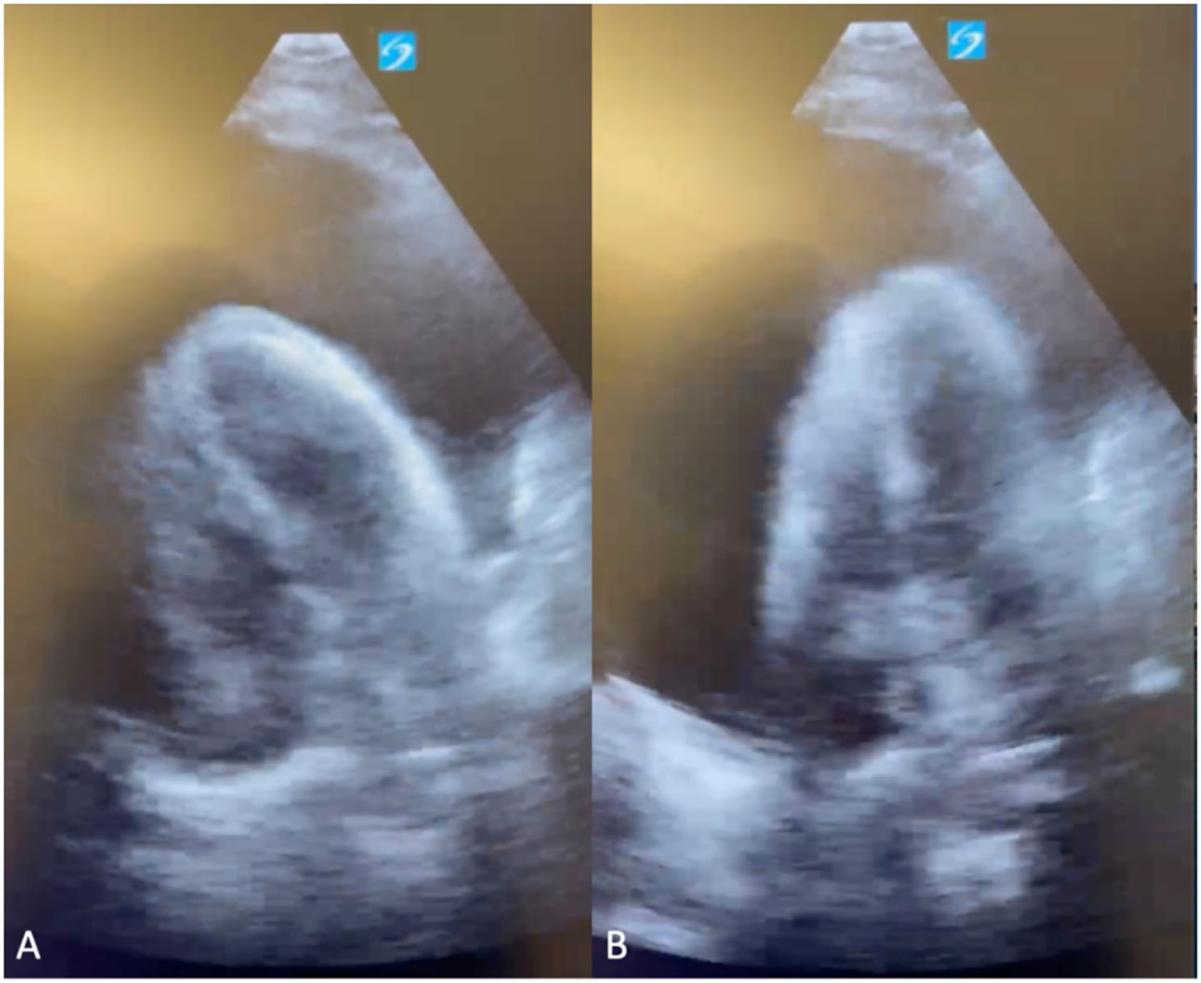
Point-of-care ultrasonography (POCUS) of large pericardial effusion. (A) POCUS demonstrates large pericardial effusion. (B) With ultrasound probe in same position as 1A, demonstrated swinging of heart within pericardial effusion
